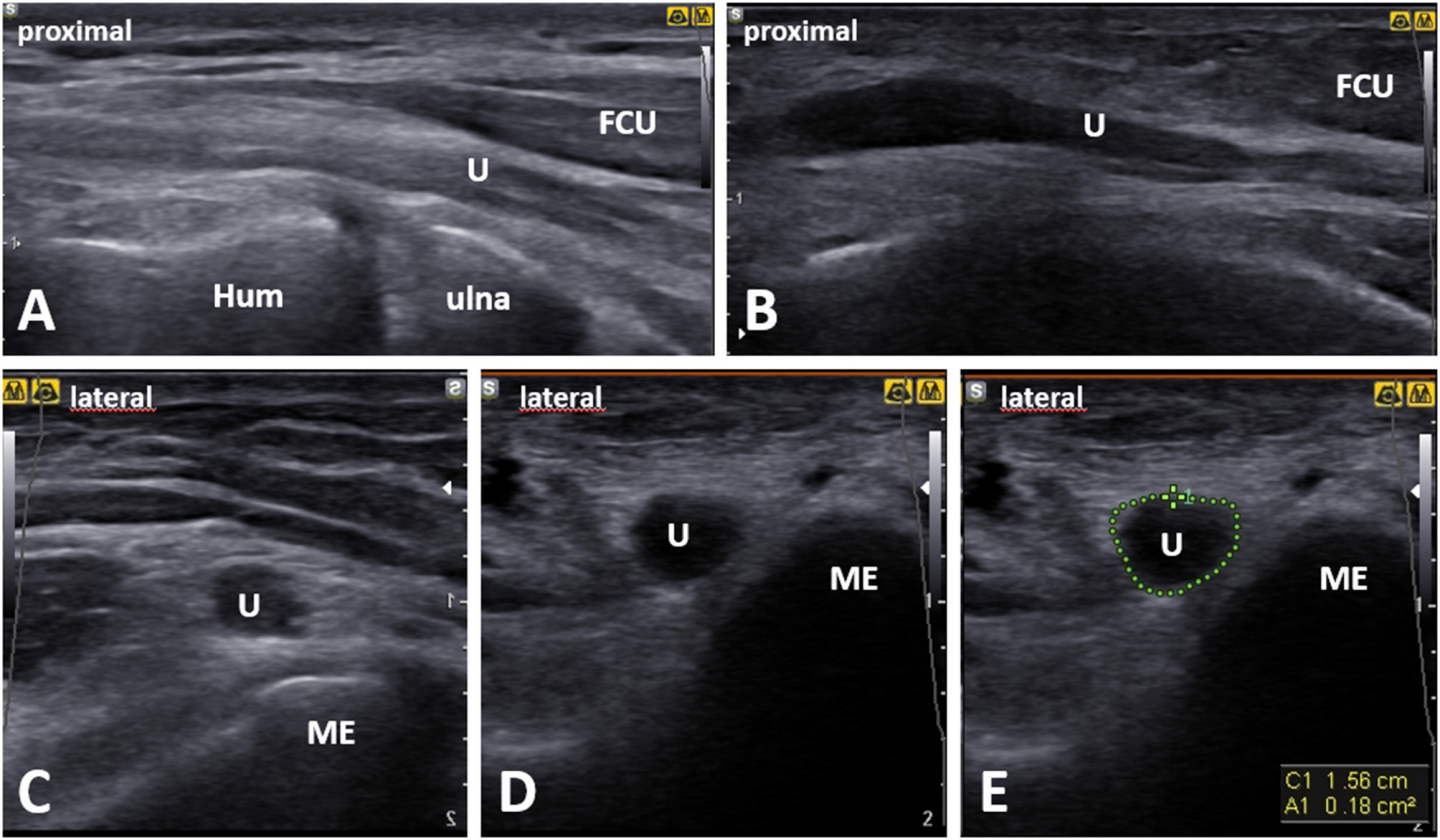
Comparative ultrasound (*US*) imaging of the ulnar nerve (*U*) at the level of the medial epicondyle (*ME*) in a patient with cubital tunnel syndrome. When compared with the normal side. (A) The asymptomatic side in a long axis of the ulnar nerve. (B) The symptomatic side ulnar nerve shows swelling (“bottle neck appearance”) proximal to the cubital tunnel inlet in long-axis. (D,E) In short axis, compared with the normal side (C), the ulnar nerve on the symptomatic side shows enlargement in its cross-sectional area of 18 mm^2^ outlined using the direct US tracing method (*green dotted line*). Hum, humerus; FCU, flexor carpi ulnaris muscle.
